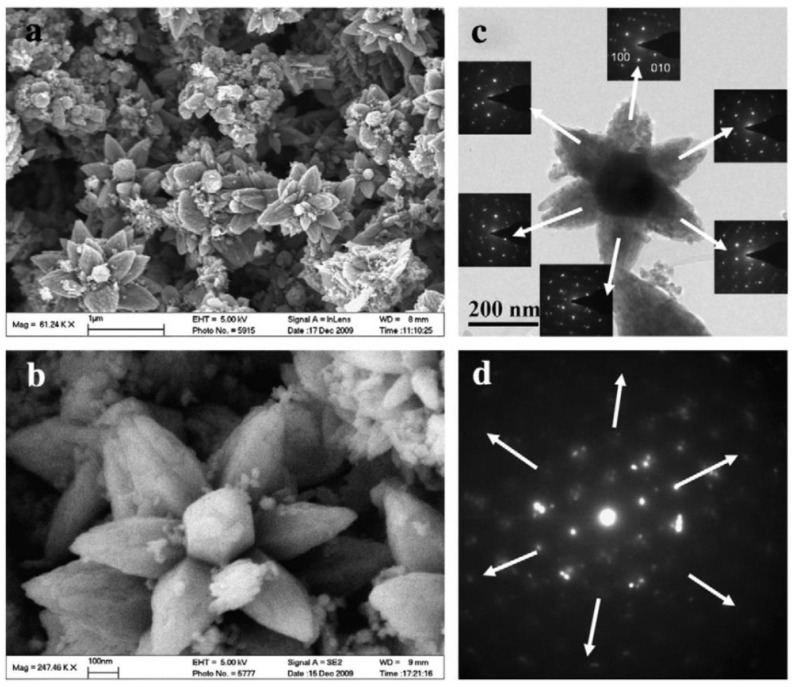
SEM images (a,b), TEM image (c) and SAED patterns (d and insets of c) of the flower-like ZnO nanostructures. Products synthesised by the microwave and ultrasonic wave combined method. Reprinted from [887], Copyright (2011), with permission from Elsevier [OR APPLICABLE SOCIETY COPYRIGHT OWNER]. All rights reserved. In order to re-use permission must be obtained from the rightsholder.
